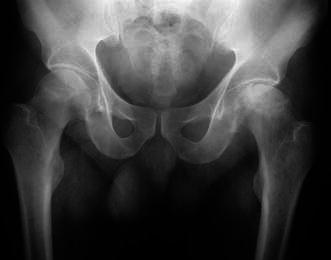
Preoperative radiograph: bilateral avascular necrosis of the femoral head
